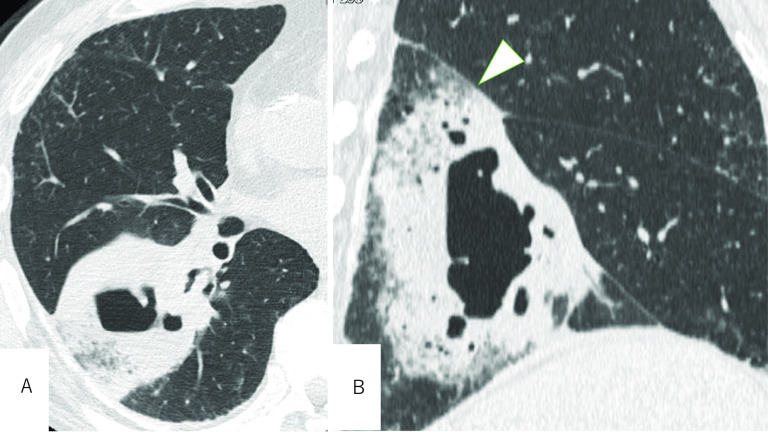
A An additional CT 6 weeks later showed a distended cavity formation. B A sagittal view revealed the abscess cavity occupying the entire lower lobe adjacent to the oblique fissure (arrowhead)
